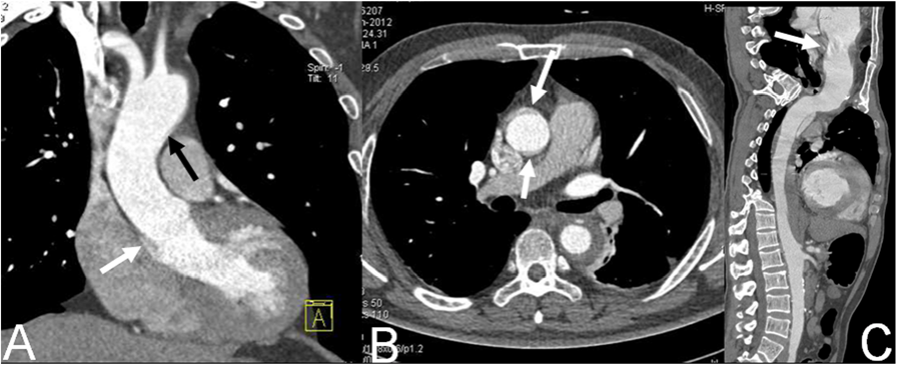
Dual-source CT imaging with traditional mode. Coronal MPR image (A) shows pulsation artifact of the aortic wall of distal ascending aorta (black arrow) and aortic sinus (white arrow). Axial original image (B) and CPR image (C) show the pulsation artifact of the ascending aortic wall (white arrows).
